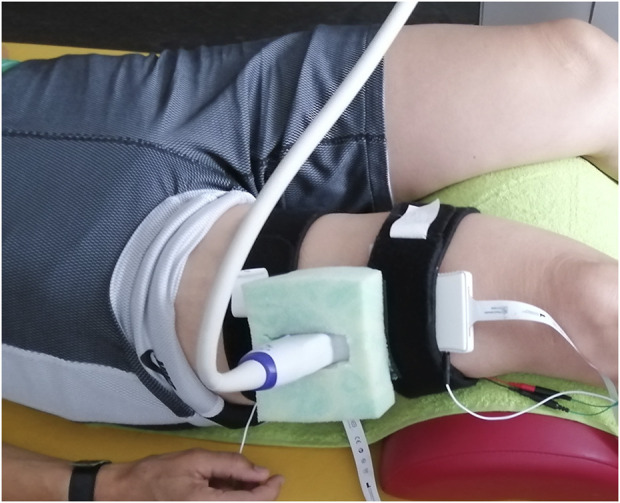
The ultrasound transducer was hosted in a custom-cut foam block, here for recording the M. vastus lateralis.
